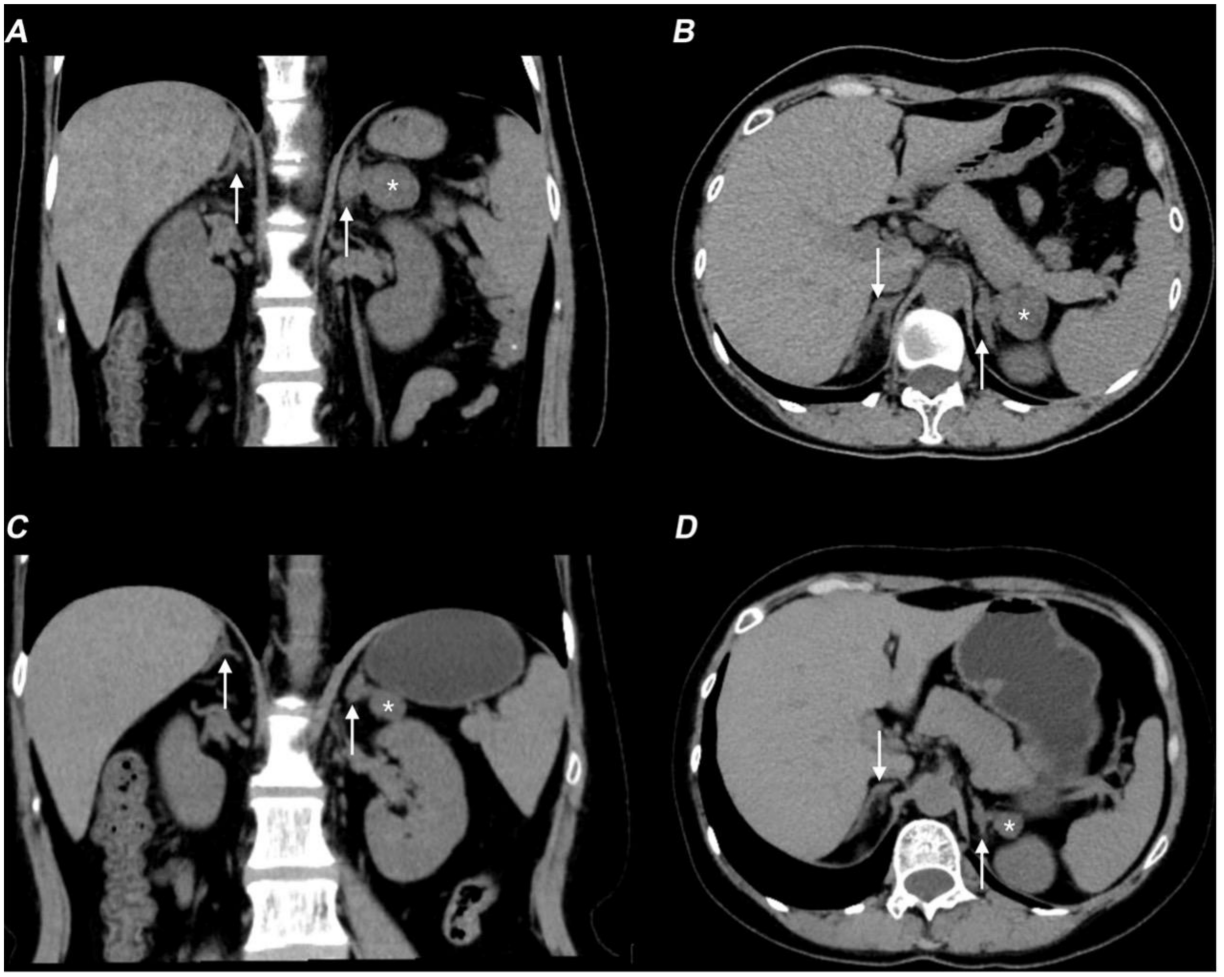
Non-contrast CT scan showing therapy-related adrenal changes in the patient with 17-OHD. (A, B) Axial (A) and coronal (B) non-contrast CT images show bilaterally enlarged adrenal glands (arrows) and a lesion (*) in the adrenal gland before therapy. (C, D) Axial (C) and coronal (D) non-contrast CT images show improvement in bilateral adrenal gland thickening (arrows) and a decrease in the size of the left adrenal mass (*).
